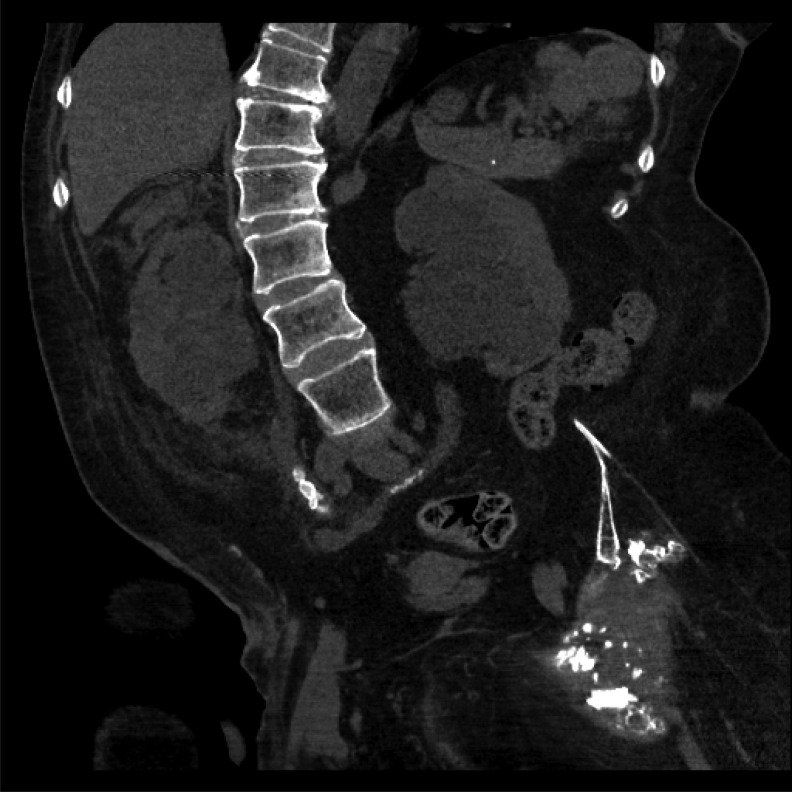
CT scan demonstrating bilateral hydroureteronephrosis, perinephric stranding and bilateral obstructing ureteral stones.
